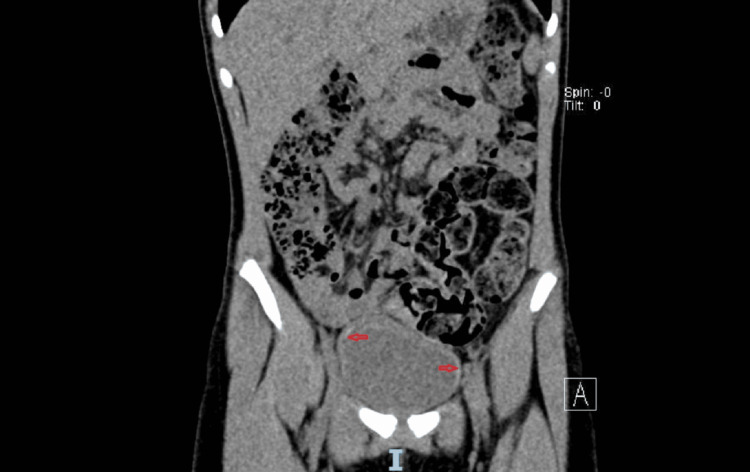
CT urography demonstrates thin, patchy calcifications along the inner bladder wall, consistent with submucosal calcifications (red arrows).
